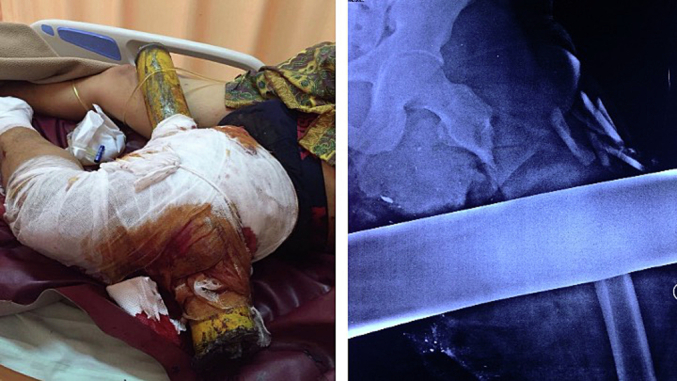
Clinical appearance of the left thigh patient and the left femur x-ray showed a displaced fracture of the left femur associated with a 15 cm bone defect.
